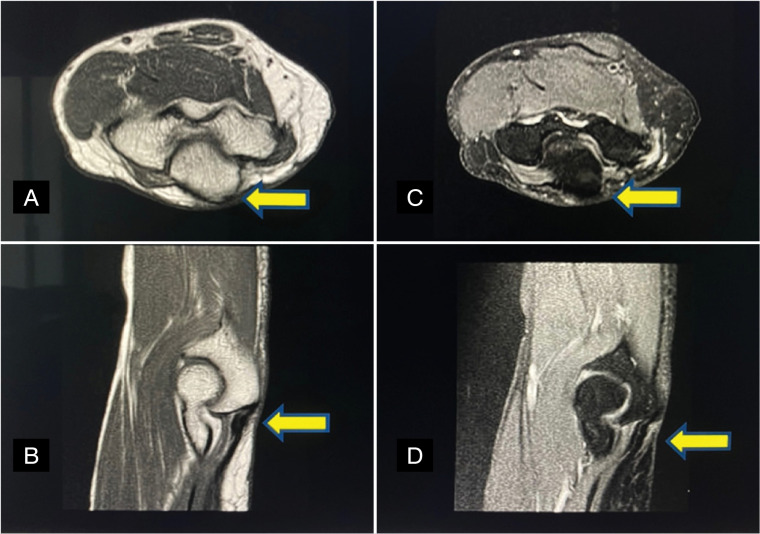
(A) and (B) T1 Sequence of axial and sagittal MRI scans show the distal triceps tendon insertion in the olecranon (yellow arrows); (C) and (D) T2 Sequence of axial and sagittal show the distal triceps insertion in the olecranon (yellow arrows).
